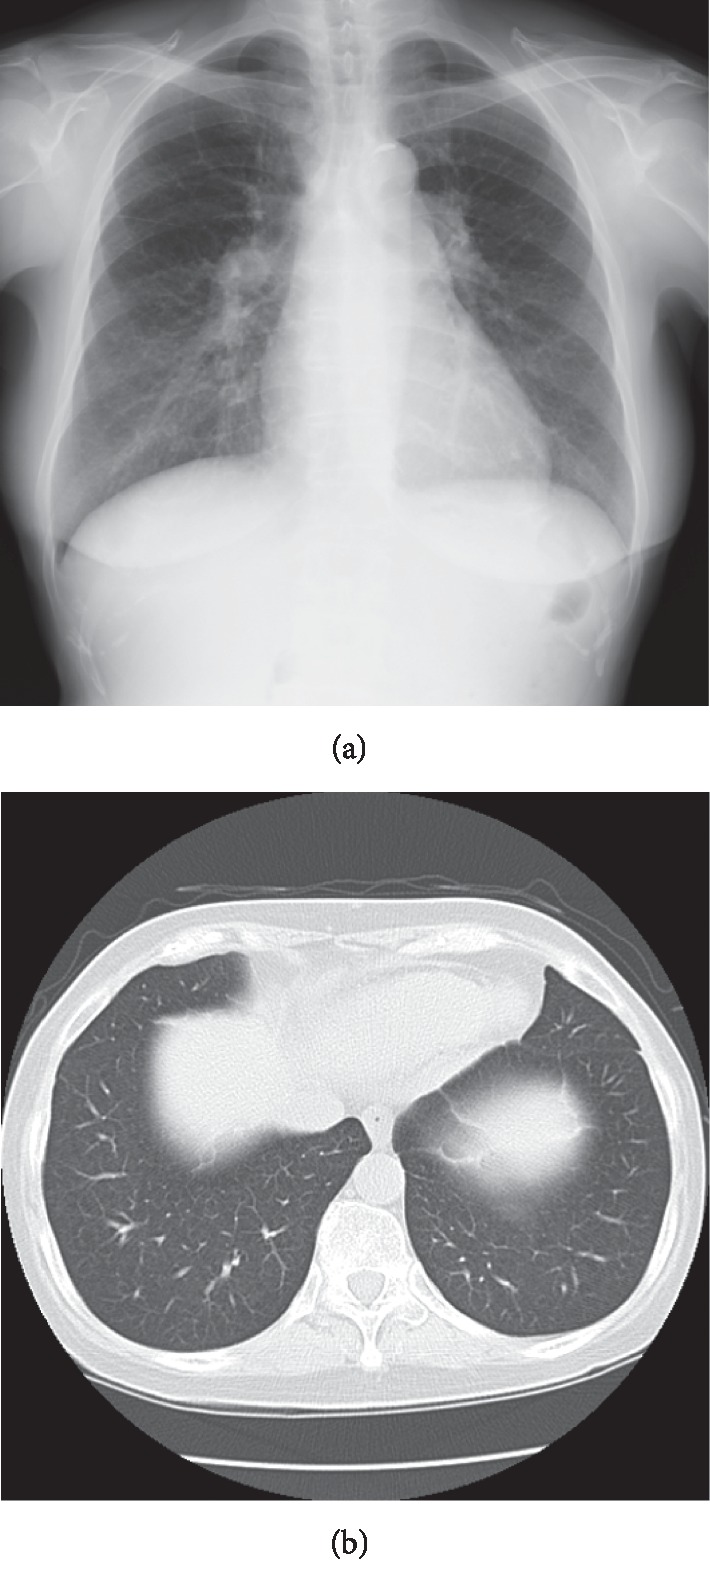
Chest X-ray and computed tomography examination performed.
